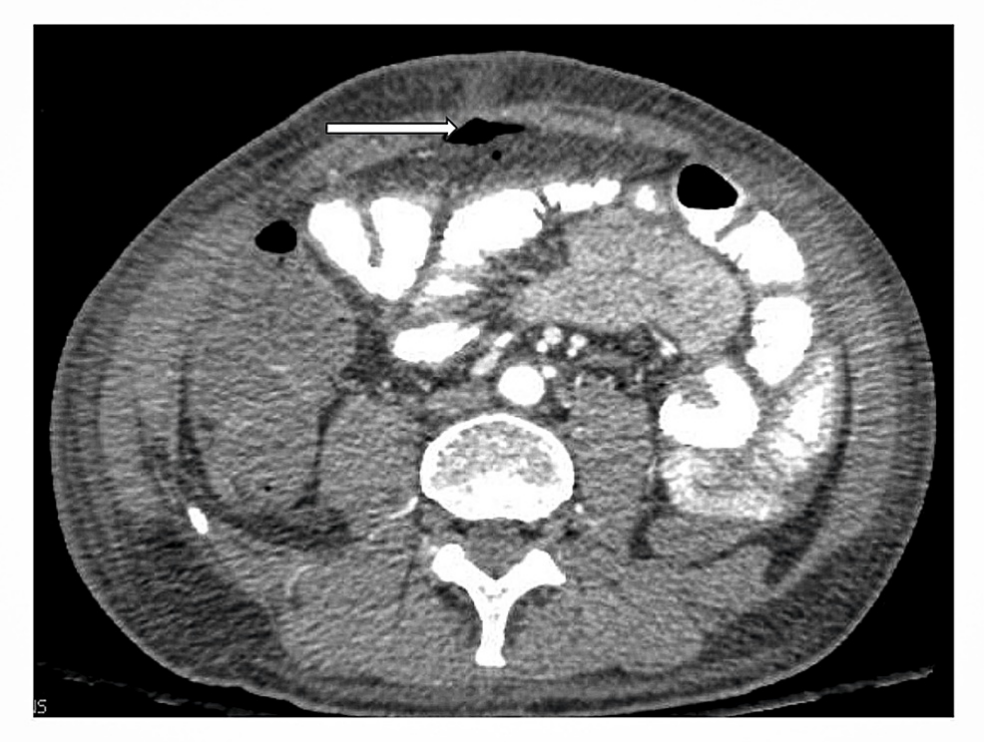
CT abdomen/pelvis with pneumoperitoneum and concern for perforation (white arrow pointing to free air in the abdomen).
